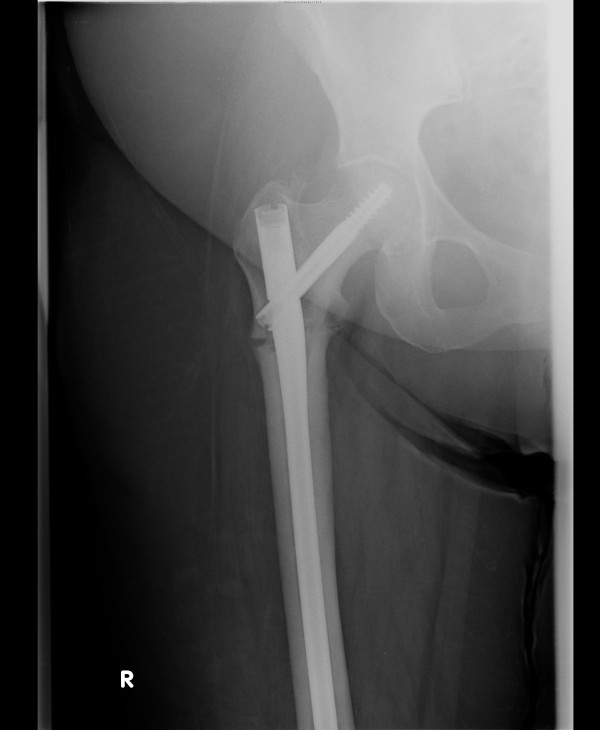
X-ray of the right hip immediately following long Gamma nail insertion.
